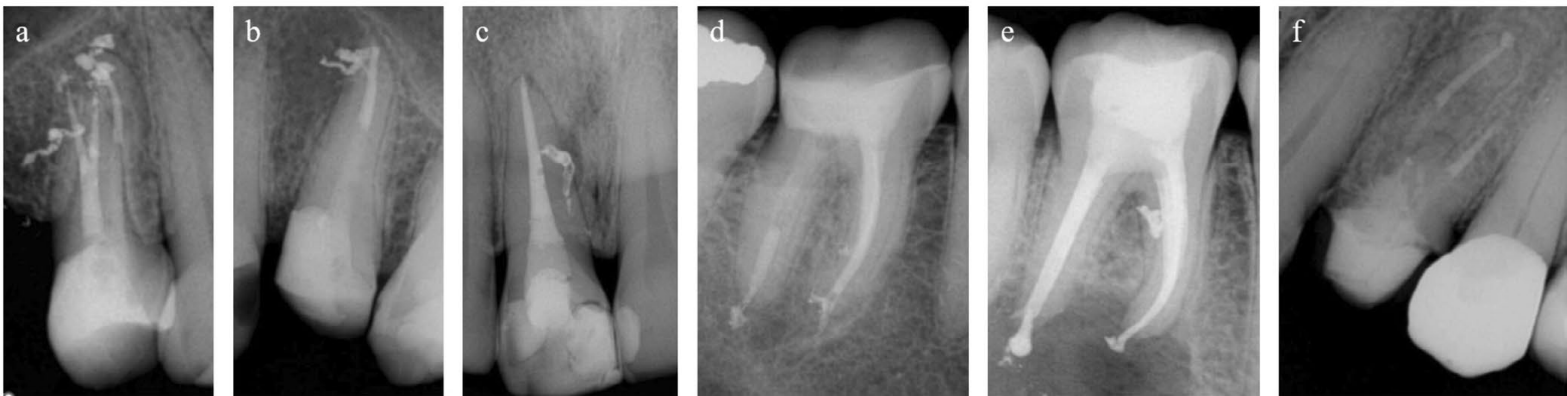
Final periapical radiograph after root canal system obturation. (a) case 1; (b) case 2; (c) case 3; (d) case 4; (e) case 5; (f) case 6.
